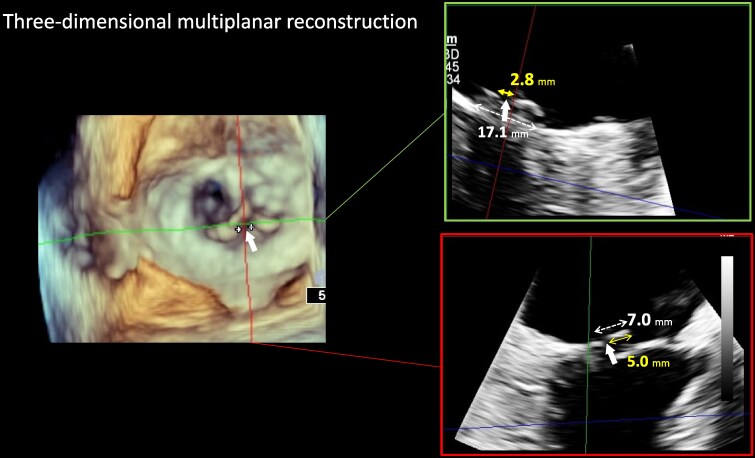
The location and size of the perforation measured by 3D multi-planar reconstruction (3d-MPR) method on transoesophageal echocardiography. The distance from the tip of P2 to the anterior edge of the perforation (white arrow) was 5.0 mm, and its posterior edge was 7.0 mm. The transverse diameter of the perforation was 2.8 mm, and the frailty width was 17.1 mm. P2: the middle segment of the posterior leaflet.
